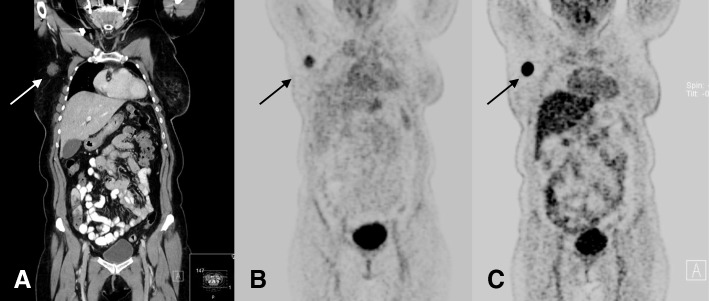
67-year-old patient with a nodal recurrence 22 months after treatment of a primary breast cancer. Coronal CT reconstruction shows a contrast enhancing lymph node metastasis with a diameter of 2.1 cm in the right axillary region (a). The lesion is visually detectable on ^68^Ga-Pentixafor PET with a corresponding SUVmax of 4.0 (b). On ^18^F-FDG PET/CT, the lesion shows a significantly higher tracer uptake (SUVmax of 24.4) (c)
